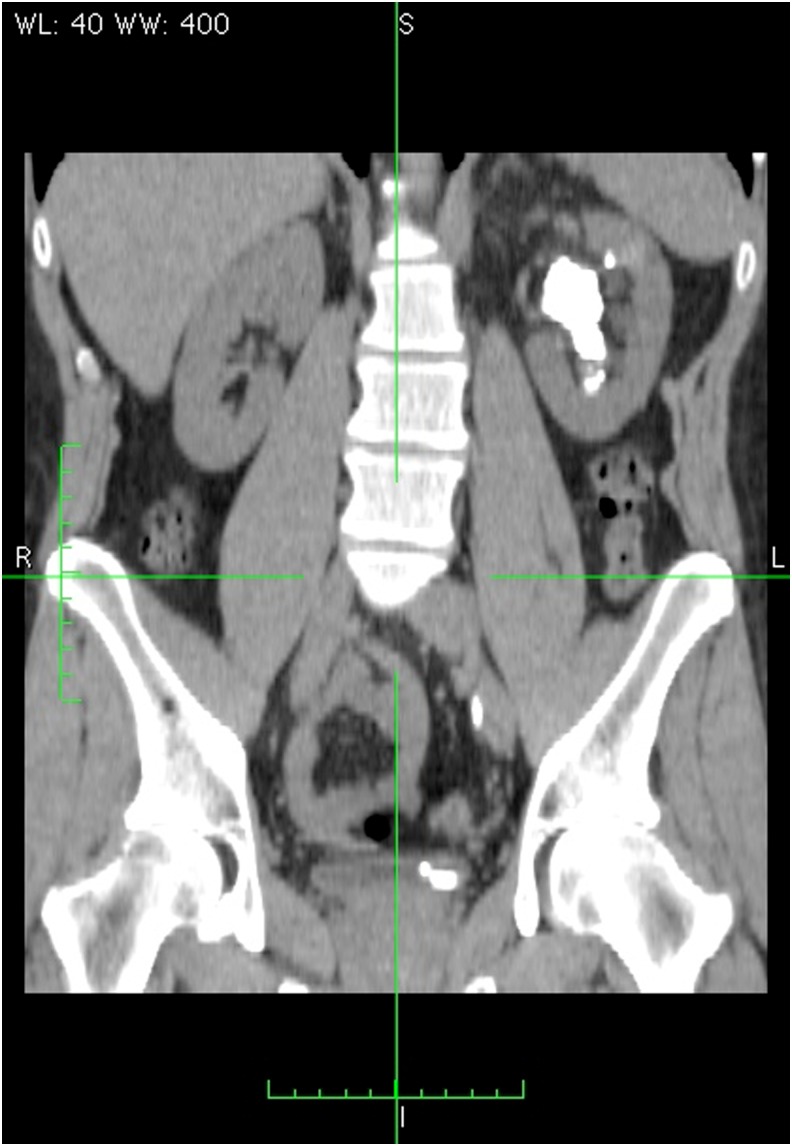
Coronal CT scan showing pretreatment staghorn left kidney stone.
